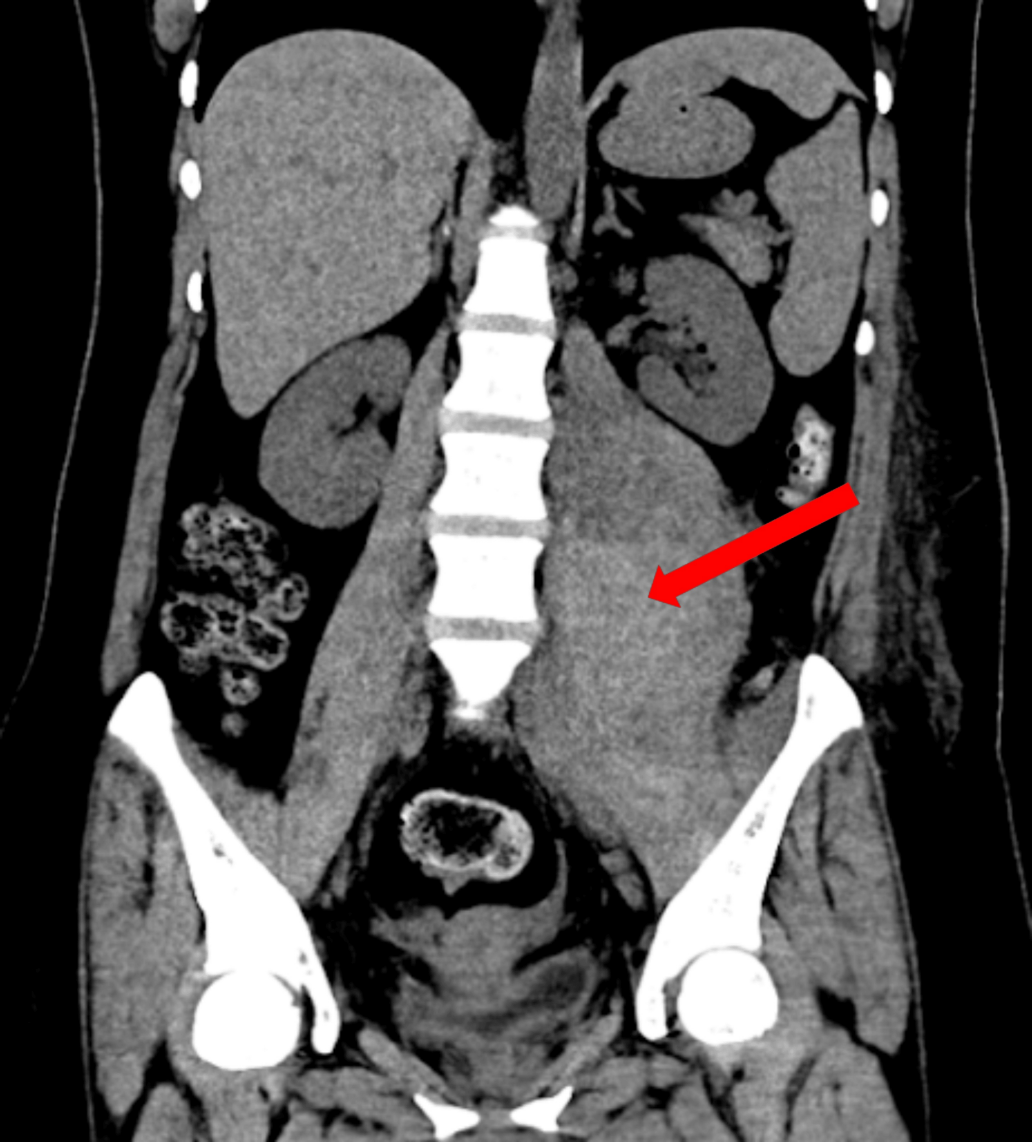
The first CT coronal reconstruction shows a heterogeneous image in the left iliopsoas muscle, consistent with a hematoma, with an estimated volume of 700 cc.
